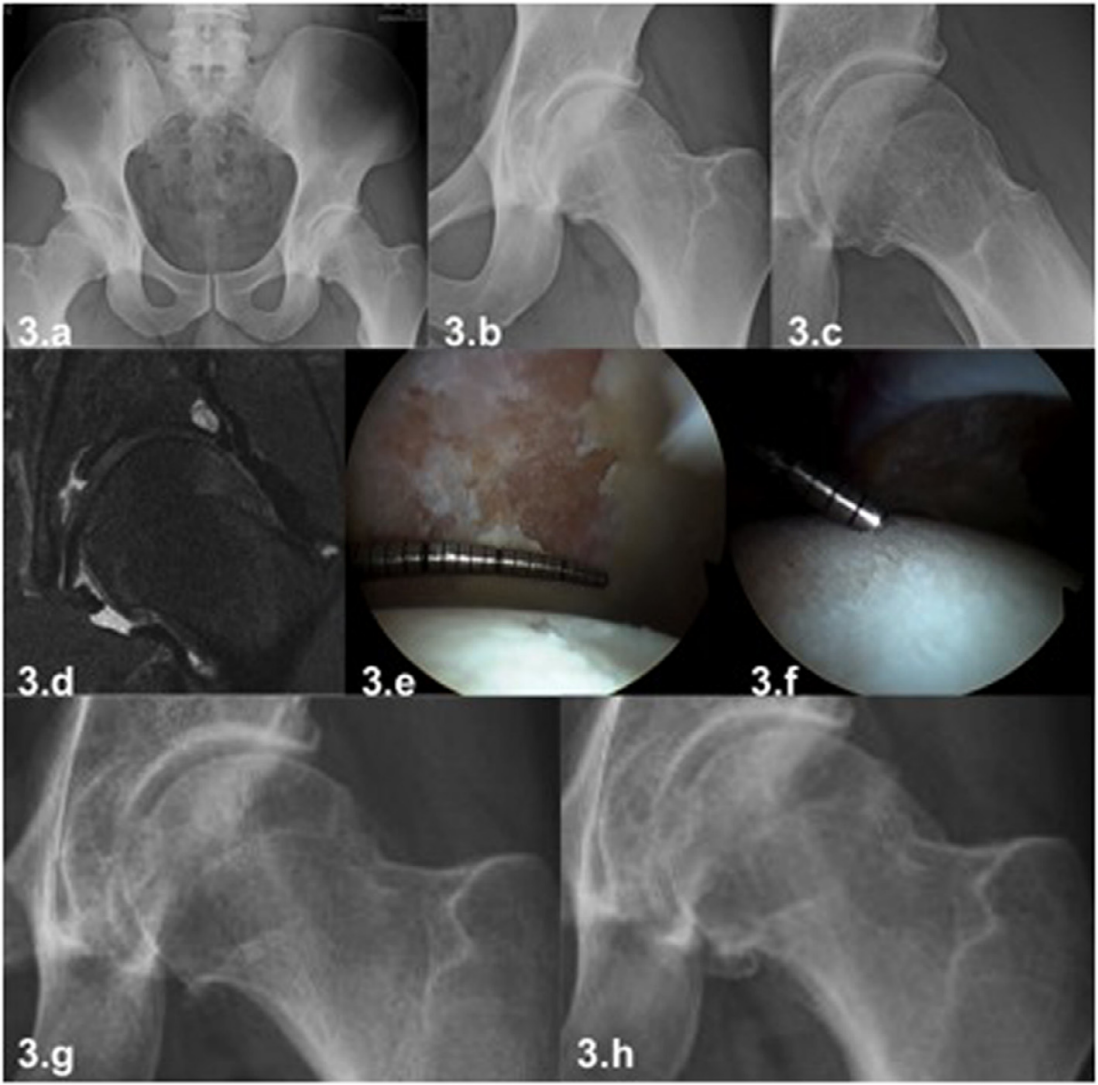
Male patient, age 32 years, intensive recreational athlete (triathlon, mountain climbing) with left hip pain in flexion and rotational movements. Clinical examination demonstrates a restriction of internal rotation in flexion above 90° associated with pain (anterior impingement). The radiograph demonstrates a FAI with a cam deformity and joint space narrowing in the peripheral joint area (3a–3c). The MRI showed a chondrolabral lesion and acetabular subchondral cyst (3d). Considering his young age and his rejection to a THR a hip arthroscopy was performed. Full-thickness chondral damage in acetabulum (3e) and diffuse fibrillation of cartilage in femoral head was found during the arthroscopy (3f). These should be considered an advanced osteoarthritis finding with a very unpredictable clinical outcome. The radiography one year later shows the progression of the chondral damage of the hip (3g). This case shows that with hip arthroscopy a temporary relief of pain can be achieved in cases of advanced chondral damage but it cannot change the course of the disease and progression to osteoarthritis of the hip.
